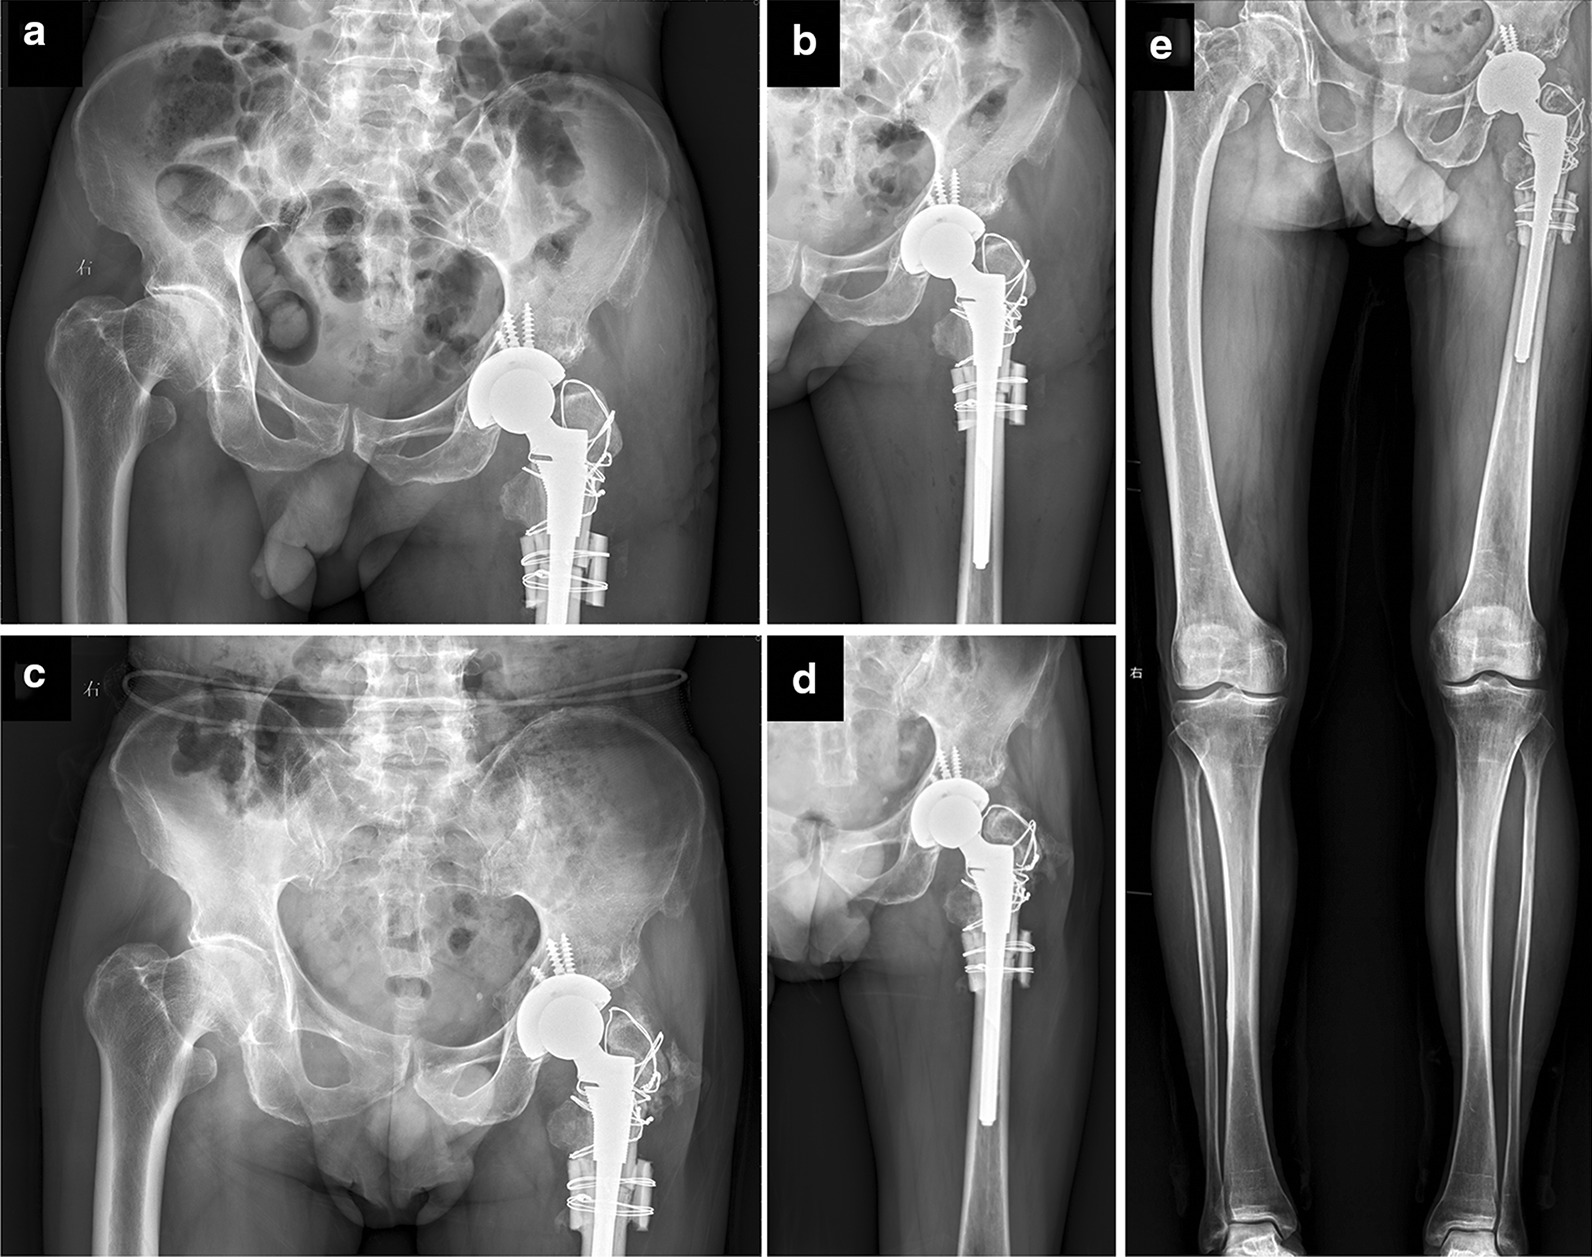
Anteroposterior radiographs of the pelvis and left hip joint at 3-day postoperatively (a and b) and 10-month follow-up postoperatively (c and d). e Full length weight bearing radiograph of lower extremities at 10-month follow-up postoperatively
